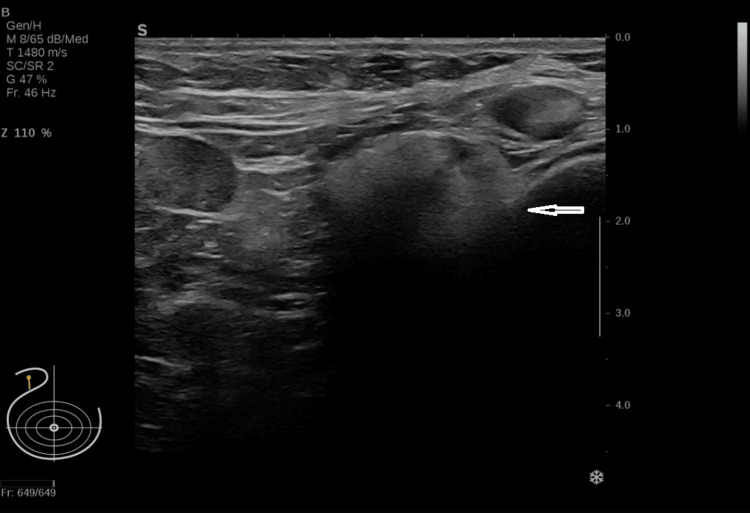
Targeted ultrasound of the right axilla demonstrating a characteristic “snowstorm” appearance, a sonographic finding consistent with silicone lymphadenopathy.
